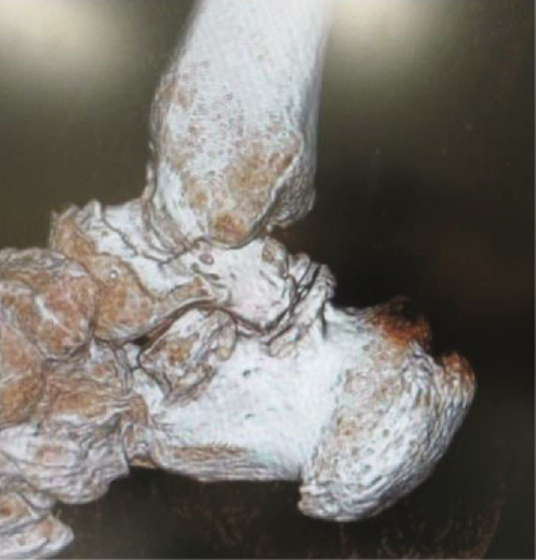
Three-dimensional computed tomography scan obtained postoperatively shows no ossified lesions in the posterior ankle.
